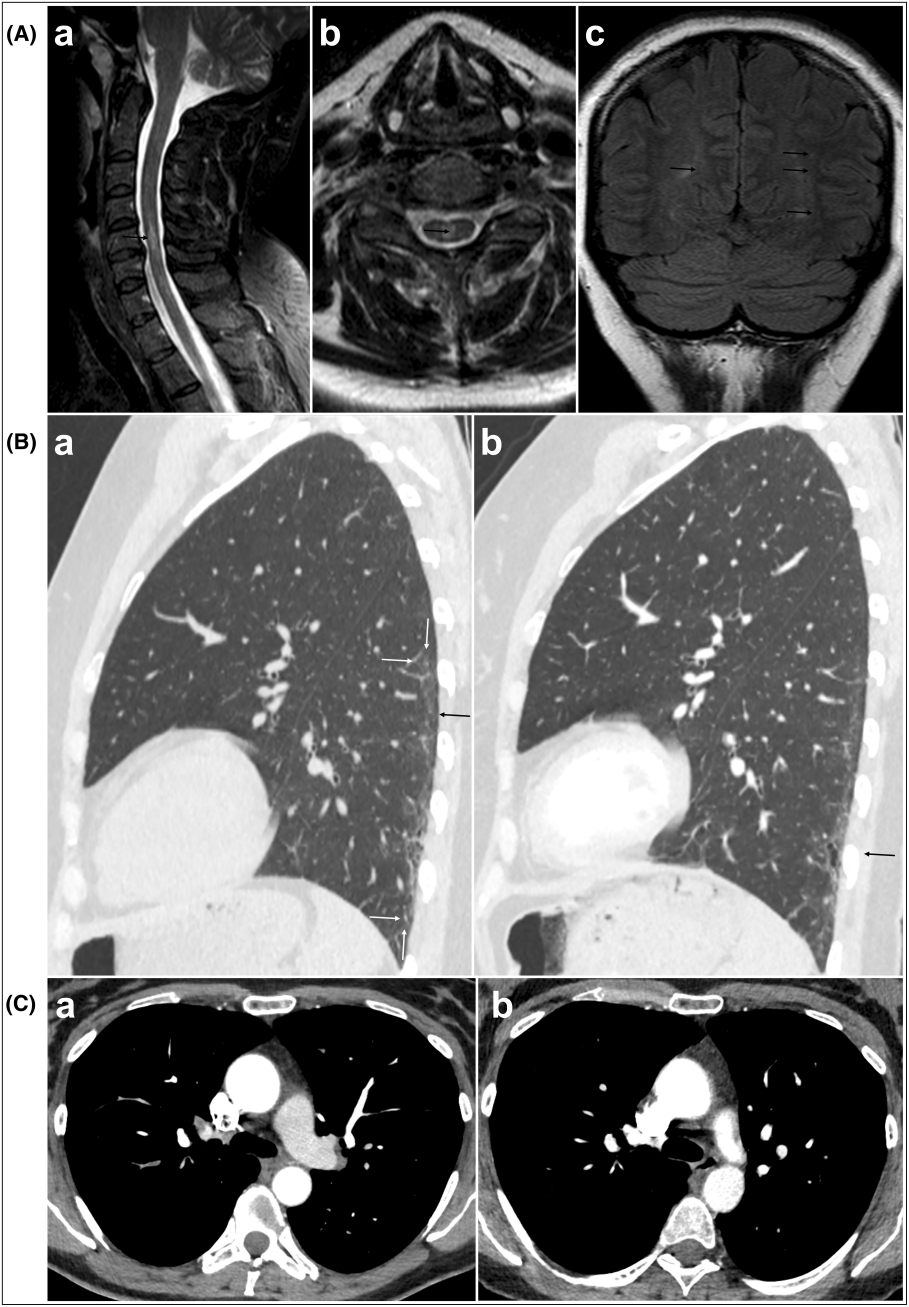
(A) Central nervous system involvement in neuromyelitis optica. (a) Cervical spine T2‐weighted sagittal and (b) axial MRI image shows short transverse myelitis with high T2 signal (arrows). (c) Brain coronal fluid attenuated inversion recovery (FLAIR) image shows some demyelinating lesions with high signal. (B) Nonspecific interstitial pneumonia (NSIP) pattern. (a) Sagittal CT image shows ground glass opacities with basal peripheral predominance (between white arrows) and subleural sparing (black arrow). (b) Three months later, the extent of the ground glass opacities is minimally reduced. Arrows indicate traction bronchiectasis. (C) Acute pulmonary thromboembolism. (a) Axial contrast‐enhanced CT scan shows embolus in a segmental pulmonary artery of the right upper lobe (arrow). (b) Three months later the lumen was recanalized.
